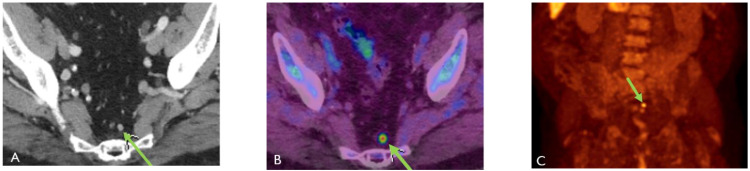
Seventy-year-old male with history of prostate cancer status post-prostatectomy, now presenting with rising PSA. (A) Contrast-enhanced CT (CECT) shows a normal-sized presacral lymph node which was presumed to be reactive. Axumin-fused PET/CT (B) and MIP (C) images show Axumin uptake within the lymph node, suspicious for recurrence and nodal disease.
